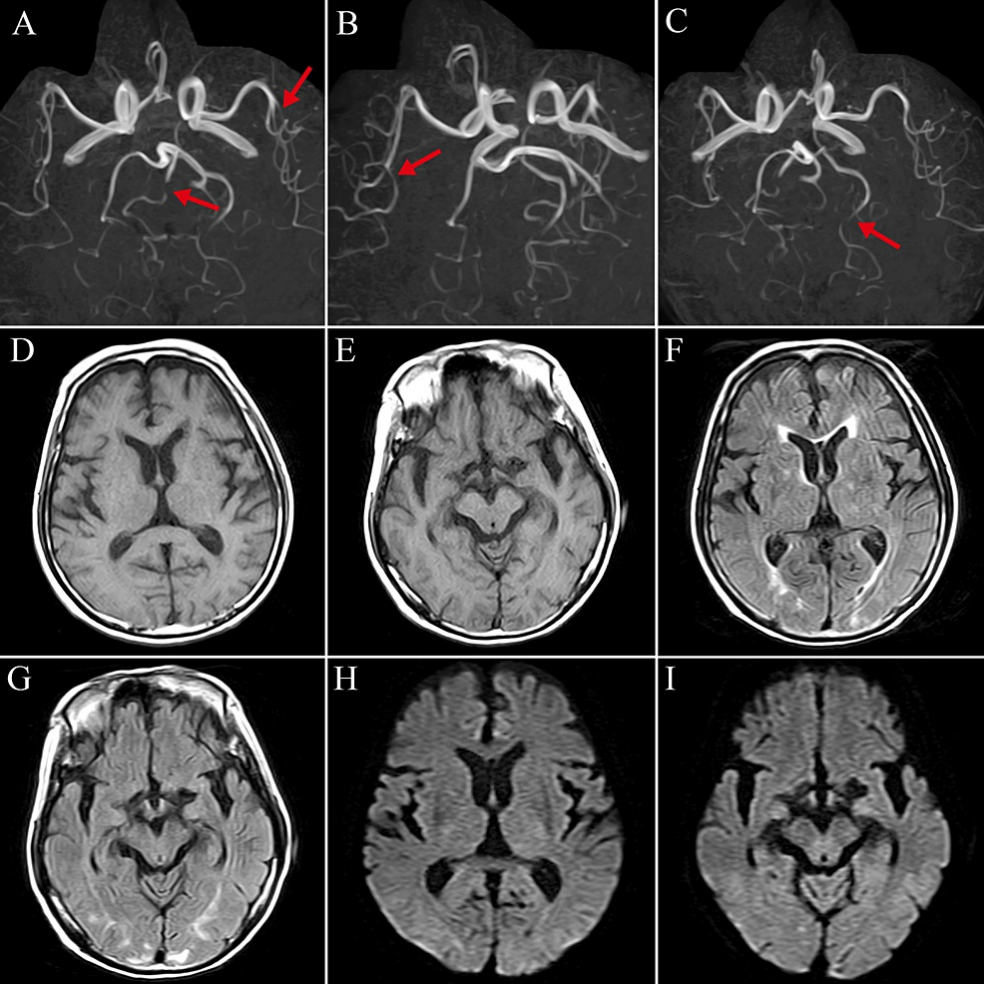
(A–C) Head magnetic resonance angiography showing multiple vasoconstrictions involving the middle and posterior cerebral arteries (red arrows). T1-weighted (D-E), FLAIR (F-G), and diffusion-weighted (H-I) images show chronic ischemic changes without any acute intracranial hemorrhage or infarction.
